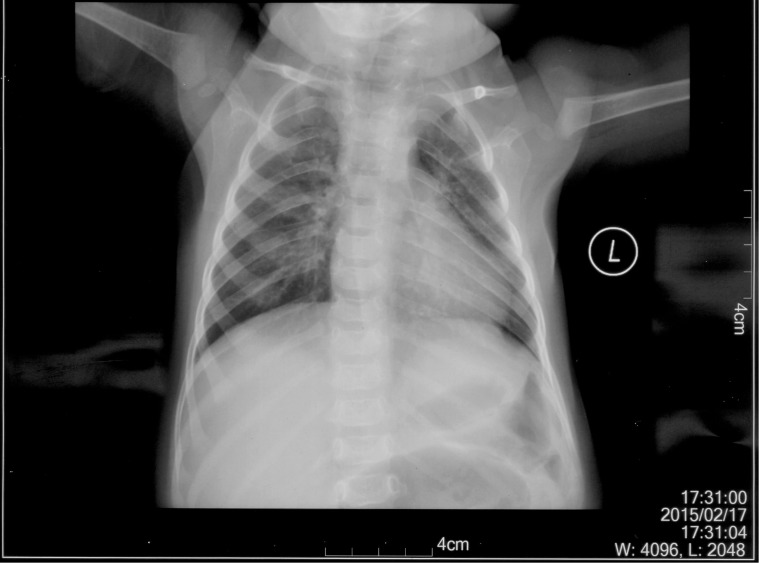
Chest CT result of the patient. It is shown that the patient had spine kyphosis.
